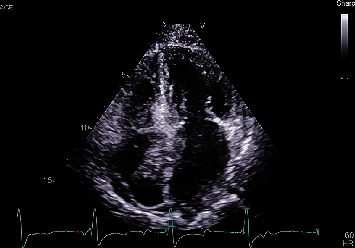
Apical four chamber from the transthoracic echocardiogram demonstrating a mass in the right atrium approximately 3-4 cm in size involving the interatrial septum.
